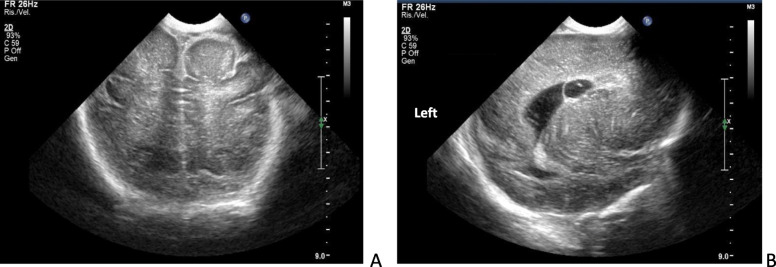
Cerebral Ultrasound images A: coronal posterior scan shows hyperechoic subcortical white matter; B: lateral sagittal scan shows ventricular pseudocysts and linear hyperechoic striae in basal ganglia
